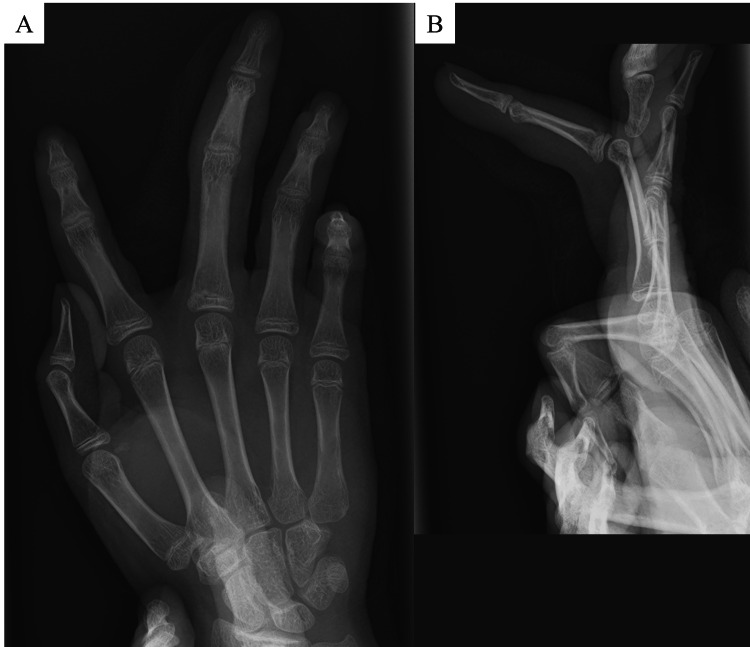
Postoperative X-ray of the right middle finger. (A) Postoperative X-ray (front view). (B) Postoperative X-ray (lateral view).
